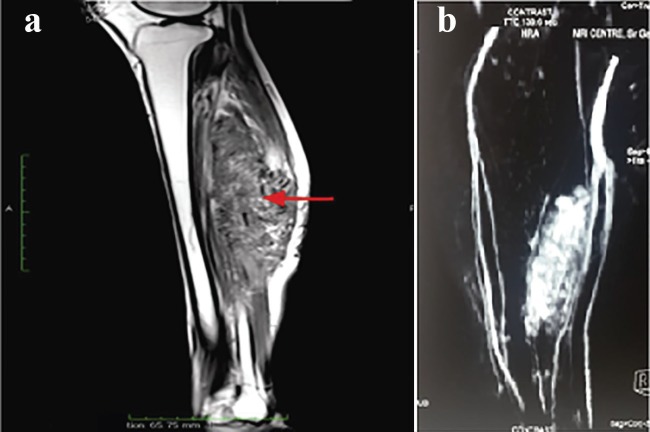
a: MRI showing arterio-venous malformation in muscle compartment. b: MR Angiography showing ossification within vascular malformation.
